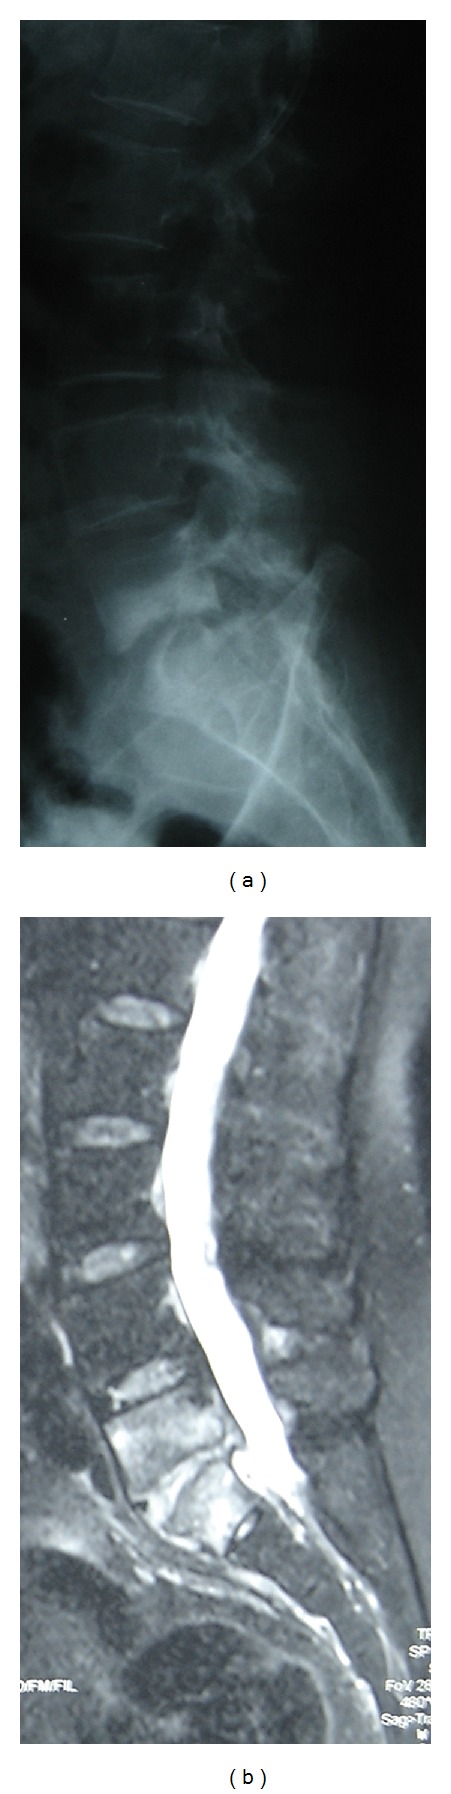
Plain films of the lumbar spine (a) and T2-weighted MR (b) showed diffuse lytic-sclerotic lesions of the vertebral bodies of L5 and S1 and disc lesions mimicking spondylodiscitis.
